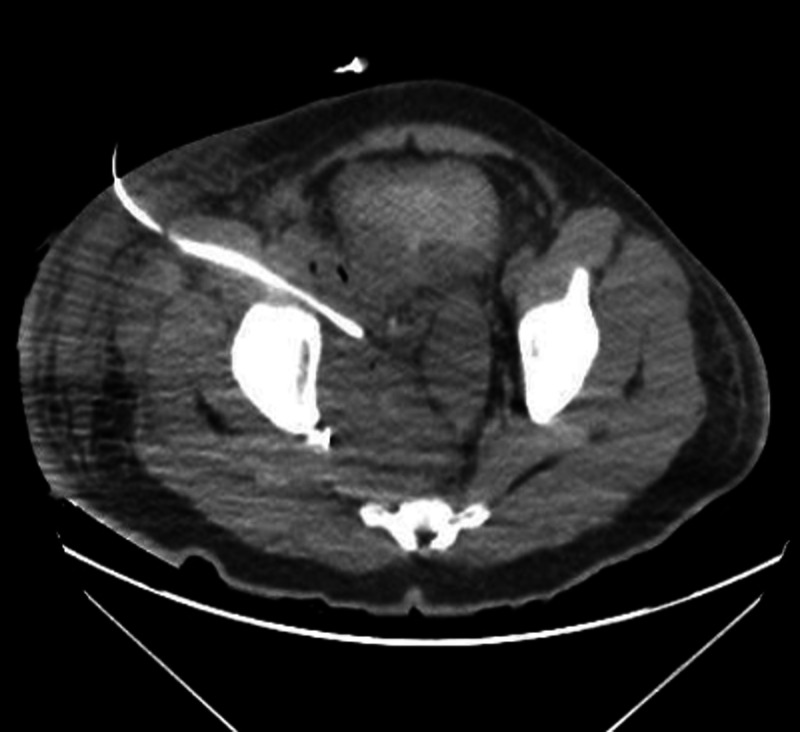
The seven-day postoperative CT scan (transverse section) displaying partial resolution of the pelvic abscess.
